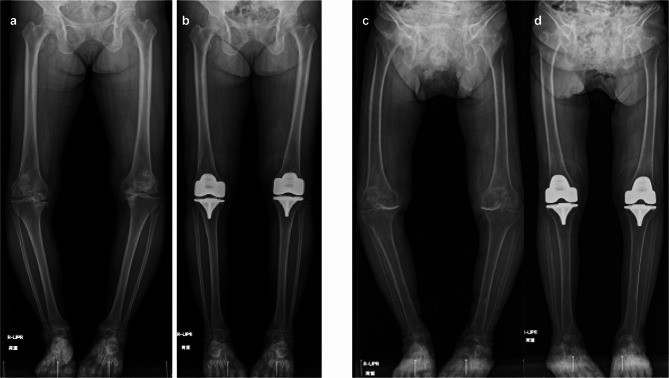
Representative pre- and postoperative radiographic findings. (a,b) Findings of a 61-year-old female. Preoperative HKAA were −21° (right) and −18° (left) varus, respectively. Postoperative HKAA are −5°(right) and −3° (left) varus, respectively. In the right knee, 9 mm of the medial bearing and 10 mm of the lateral bearing are used. In left knee, 9 mm of the medial and lateral bearing are used. (c,d) Findings of a 91-year-old female. Preoperative HKAA are −27°(right) and −22° (left) varus, respectively. Postoperative HKAA are −5°(right) and −3° (left) varus, respectively. The medial and lateral bearing of 9 mm are used in both the knees. *HKAA* hip–knee–ankle angle.
